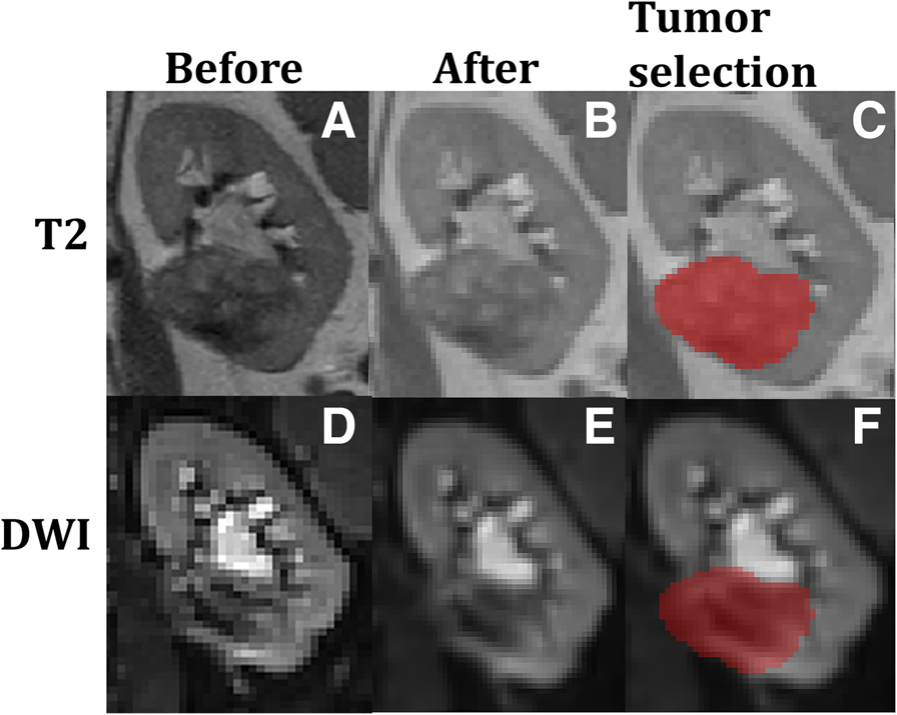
Anatomical reference *T*_*2*_ image before (a) and after (b) motion correction and image registration diffusion weighted images before (d) and after (e) processing, ROI selecting tumor tissue in *T*_*2*_ (c) and same ROI projected on DWI (f)
